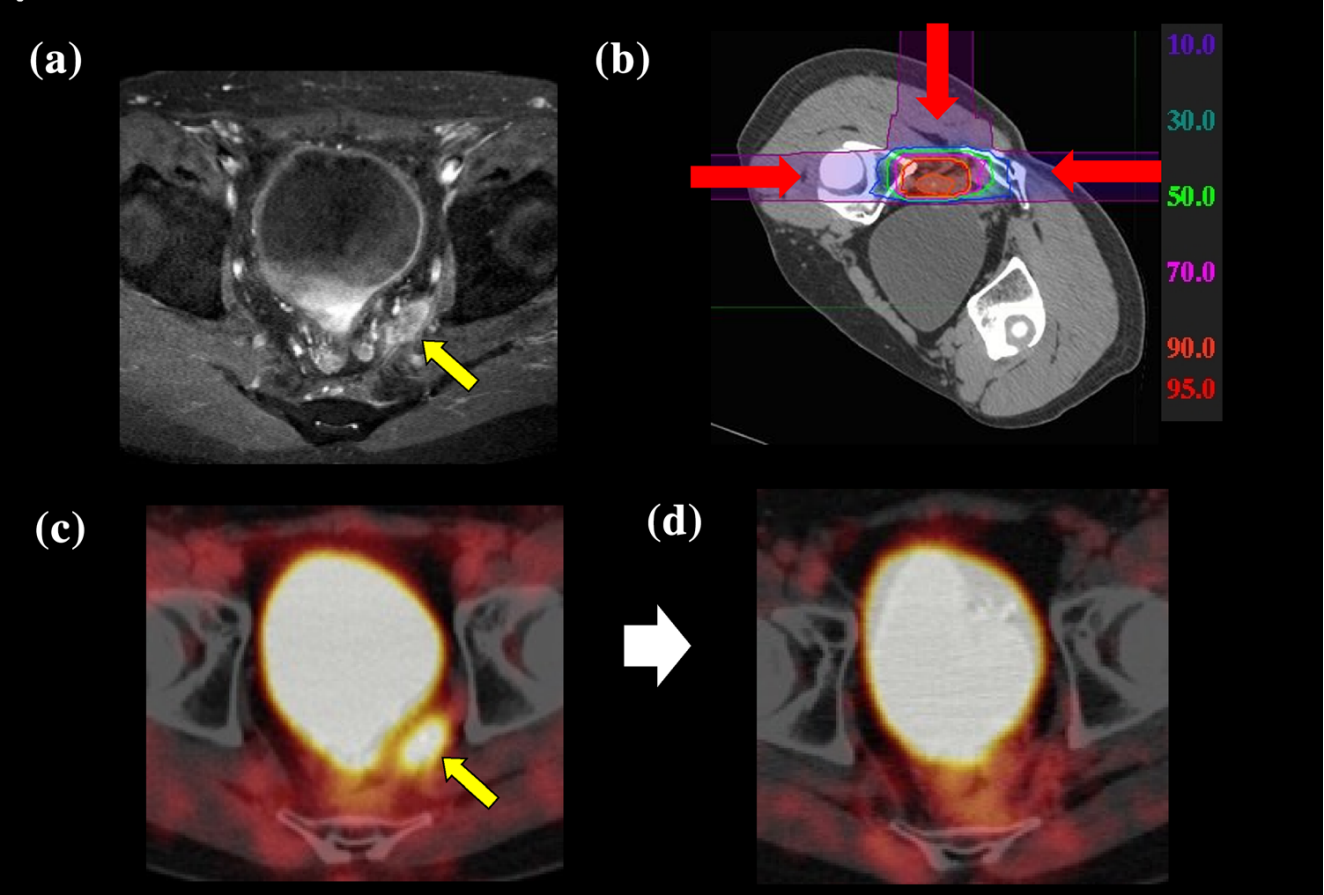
This patient had received 40 Gy X-ray treatment 17 months before carbon-ion radiotherapy (CIRT). a T1 magnetic resonance imaging before treatment showing a tumor mass in the presacral space (*yellow arrow*). b Depth-dose distribution of the carbon ion beam in recurrent rectal cancer (*red line:* 90 % isodose of the prescribed dose). c Positron emission tomography (PET) imaging before treatment. d The PET 48 months after treatment demonstrating disappearance of the left-side wall mass. At this writing, the patient is alive 8 years after CIRT with no signs of recurrence.
